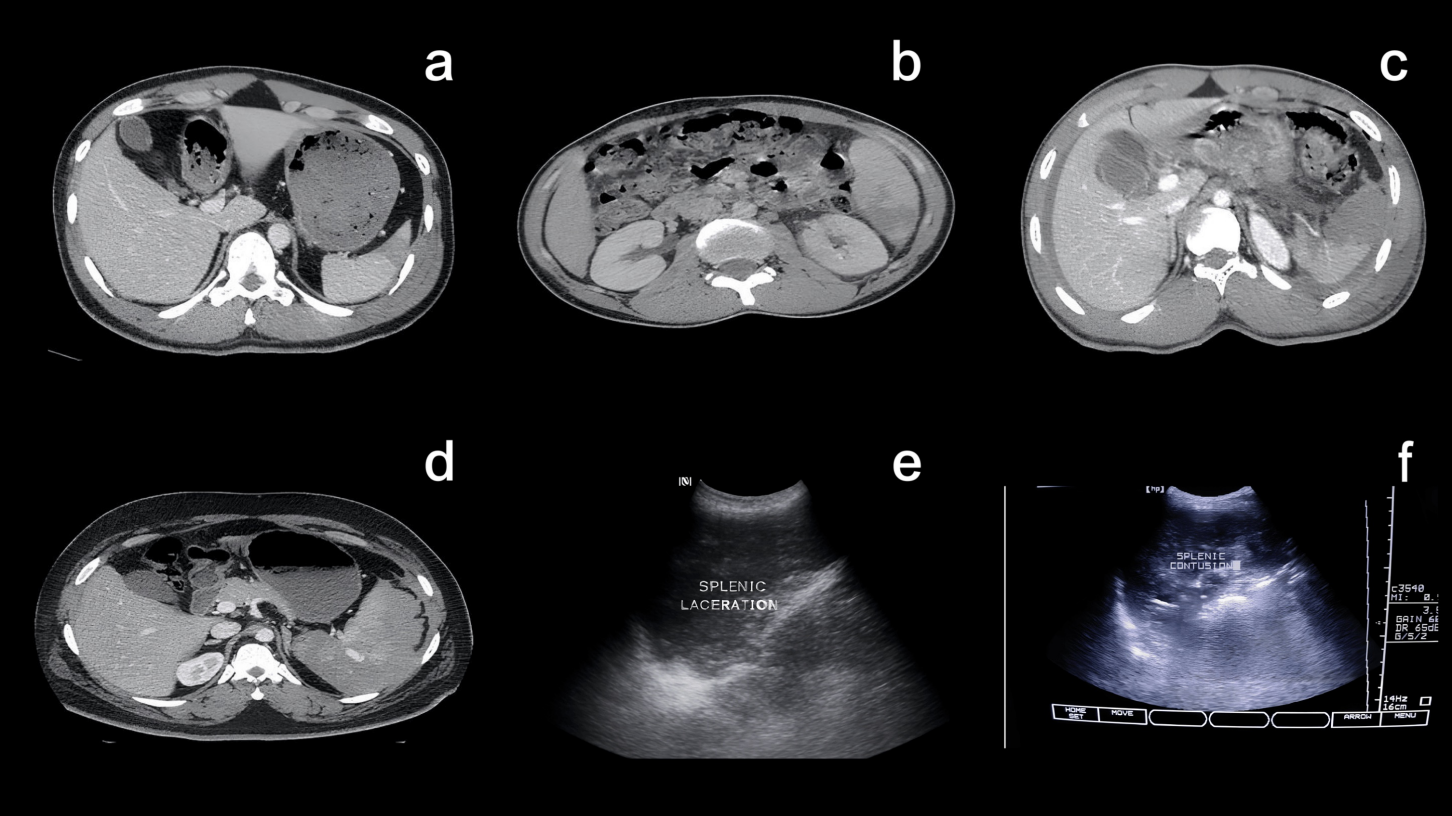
Imaging findings in splenic trauma (a) Contrast-enhanced CT axial image showing a Grade I splenic laceration with a linear hypodense defect in the parenchyma. (b) CT image demonstrating a Grade II splenic injury with deeper parenchymal disruption. (c) CT scan depicting a Grade III splenic laceration with associated perisplenic hematoma. (d) CT image of a Grade IV splenic injury showing extensive parenchymal disruption involving more than 25-50% of the splenic volume. (e) USG image showing a hypoechoic parenchymal defect consistent with splenic laceration. (f) USG image demonstrating diffuse hypoechoic changes suggestive of splenic contusion.
